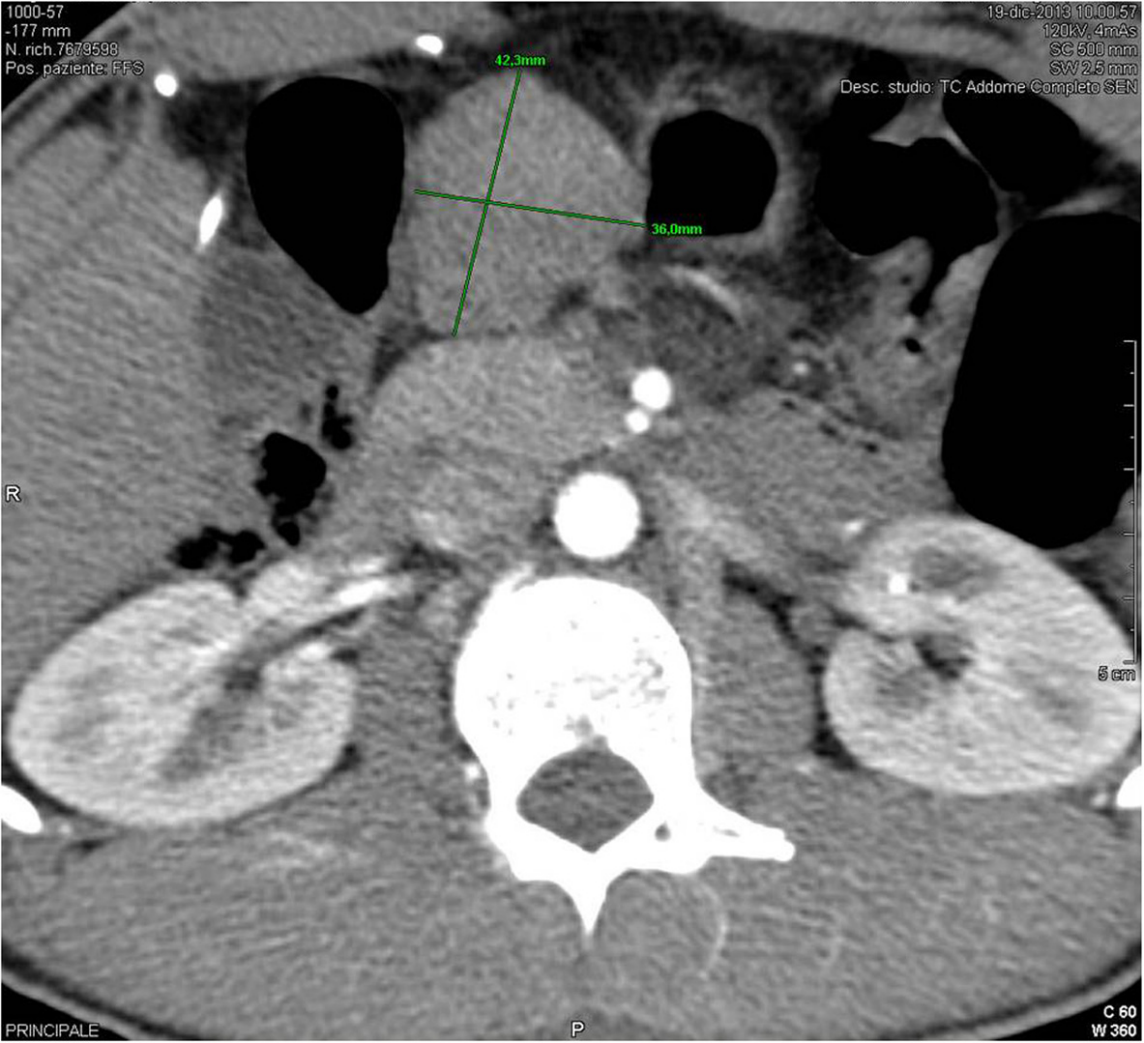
Abdominal CT-scan with contrast: solid round lesion (42×36cm) adjacent to the left liver lobe, the stomach and the pancreas.
